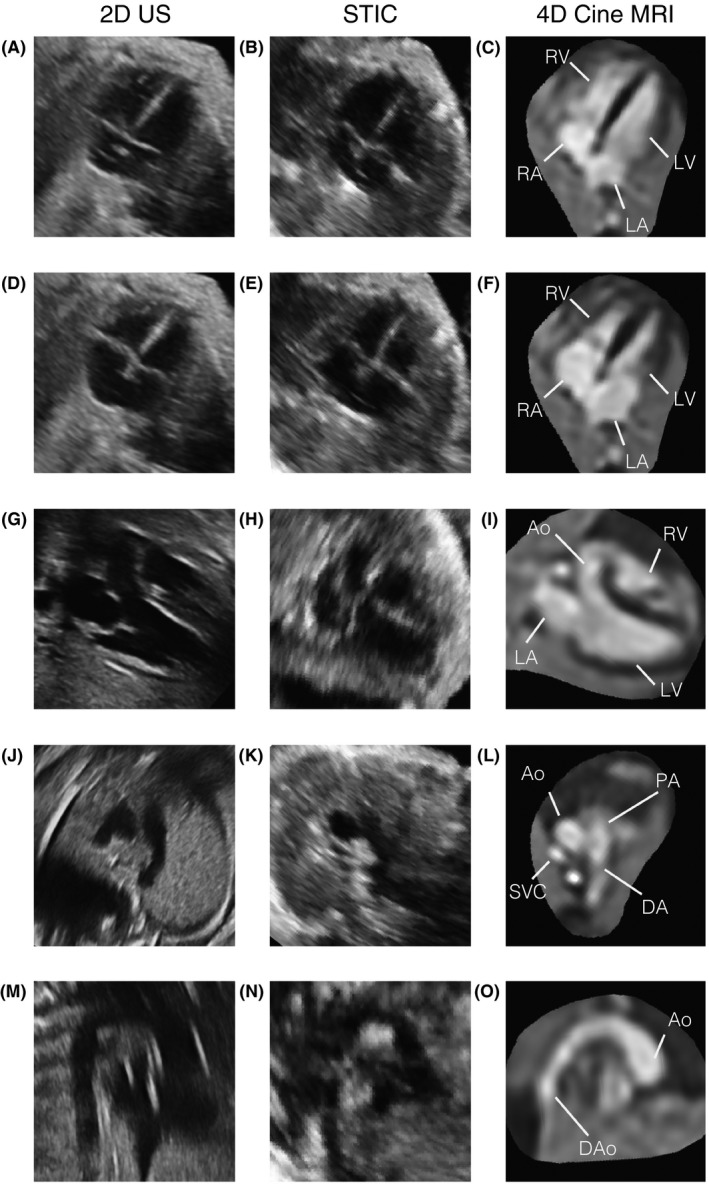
Comparison of MRI and ultrasound (US) in a $32^{+1}$ week gestational age fetus (ID01) with moderate dilation of the ascending aortic root and rightward tortuosity of the proximal ascending aorta. The fetal heart is shown in matched views in 2D echocardiogram (left column), 4D spatio‐temporal image correlation (STIC) ultrasound (centre column) and reconstructed 4D cine MRI (right column). Four‐chamber views at (A)‐(C) end‐ventricular diastole and (D)‐(F) end‐ventricular systole show the alternating contraction and expansion of the ventricles (LV, RV) and atria (LA, RA), with balanced chambers. (G)‐(I) Left 3‐chamber view, (J)‐(L) 3‐vessel view and (M)‐(O) aortic arch in sagittal view revealing a dilated aorta (Ao) at the sinotubular junction, compared to the pulmonary artery (PA), ductal arch (DA) and descending aorta (DAo). Some artefacts can be seen in the 2D US and STIC data, occasionally obscuring cardiac anatomy, while MRI had good signal coverage of the entire cardiovascular system. All bounding boxes measuring 65 × 65 mm and the fetal heart is shown in radiological orientation. The 4D MRI views are shown using spatial B‐spline interpolation to avoid voxel distortions
